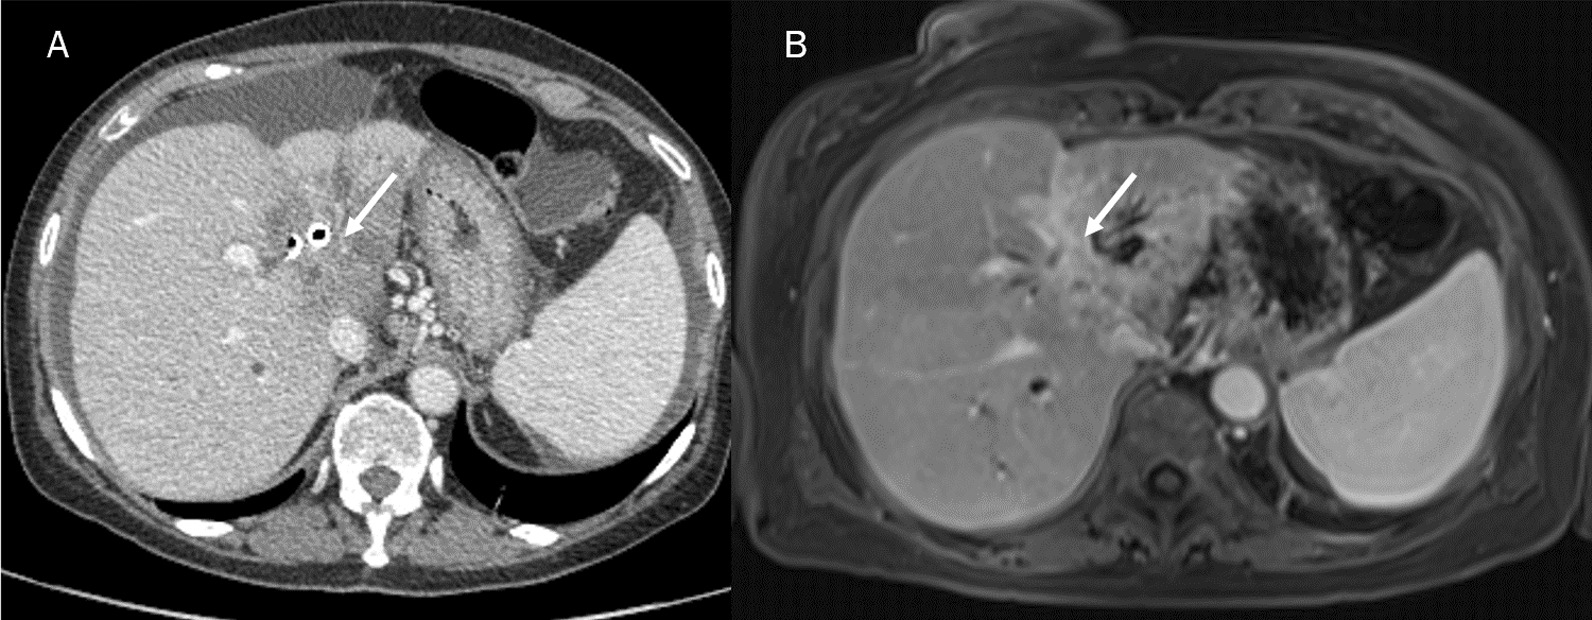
CT (A) and MRI (B) portal phase assessment of ICC. The typical contrast enhancement is a gradual centripetal enhancement on dynamic studies (arrows)
